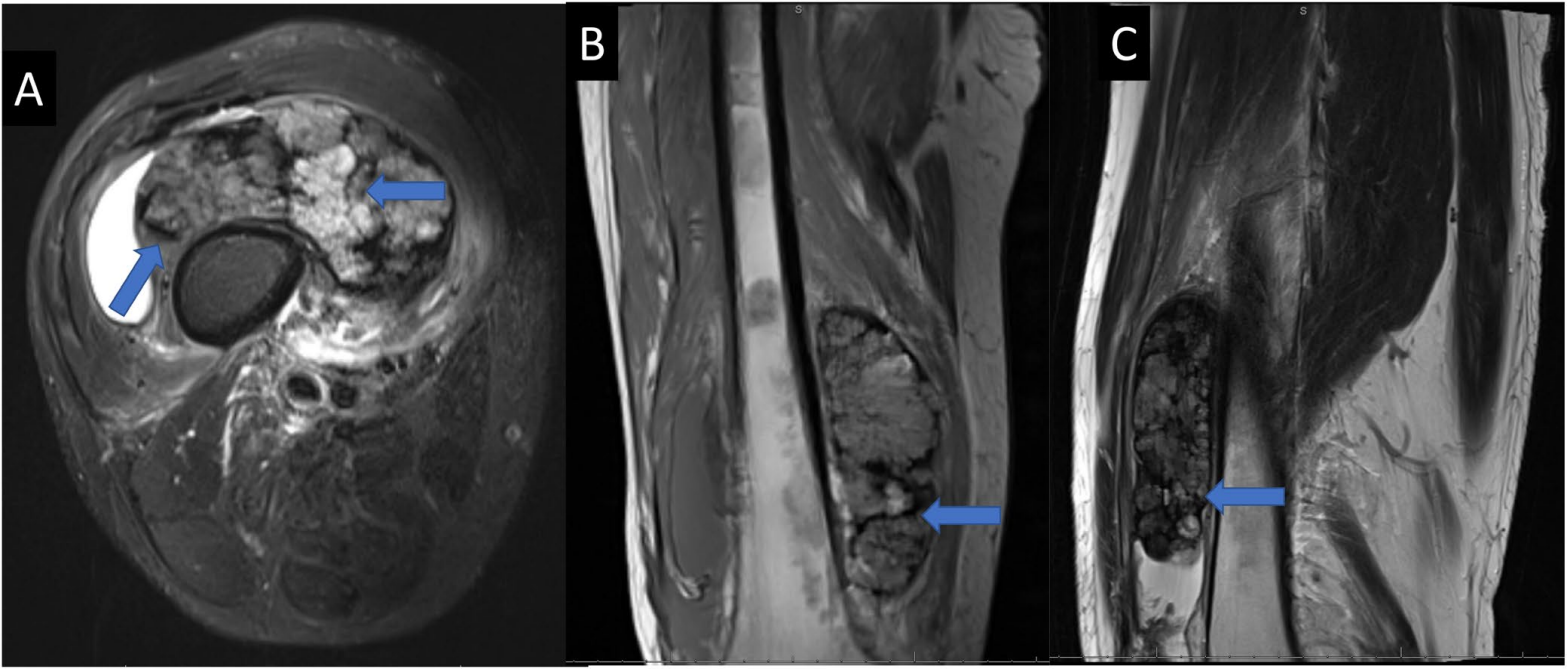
Axial T2 fat suppressed (A), coronal T1 (B) and sagittal proton density. (C) MRI demonstrates a solid extra‐skeletal extraarticular lobulated soft tissue mass, with heterogenous T1 and T2 signal intensity adjacent to the femoral diaphysis, with mild peritumoral edema. There is no evidence of cortical or medullary osseous involvement. The lesion contains internal linear and curvilinear areas of low signal intensity corresponding to hemosiderin deposition and fibrous septa (blue arrows).
